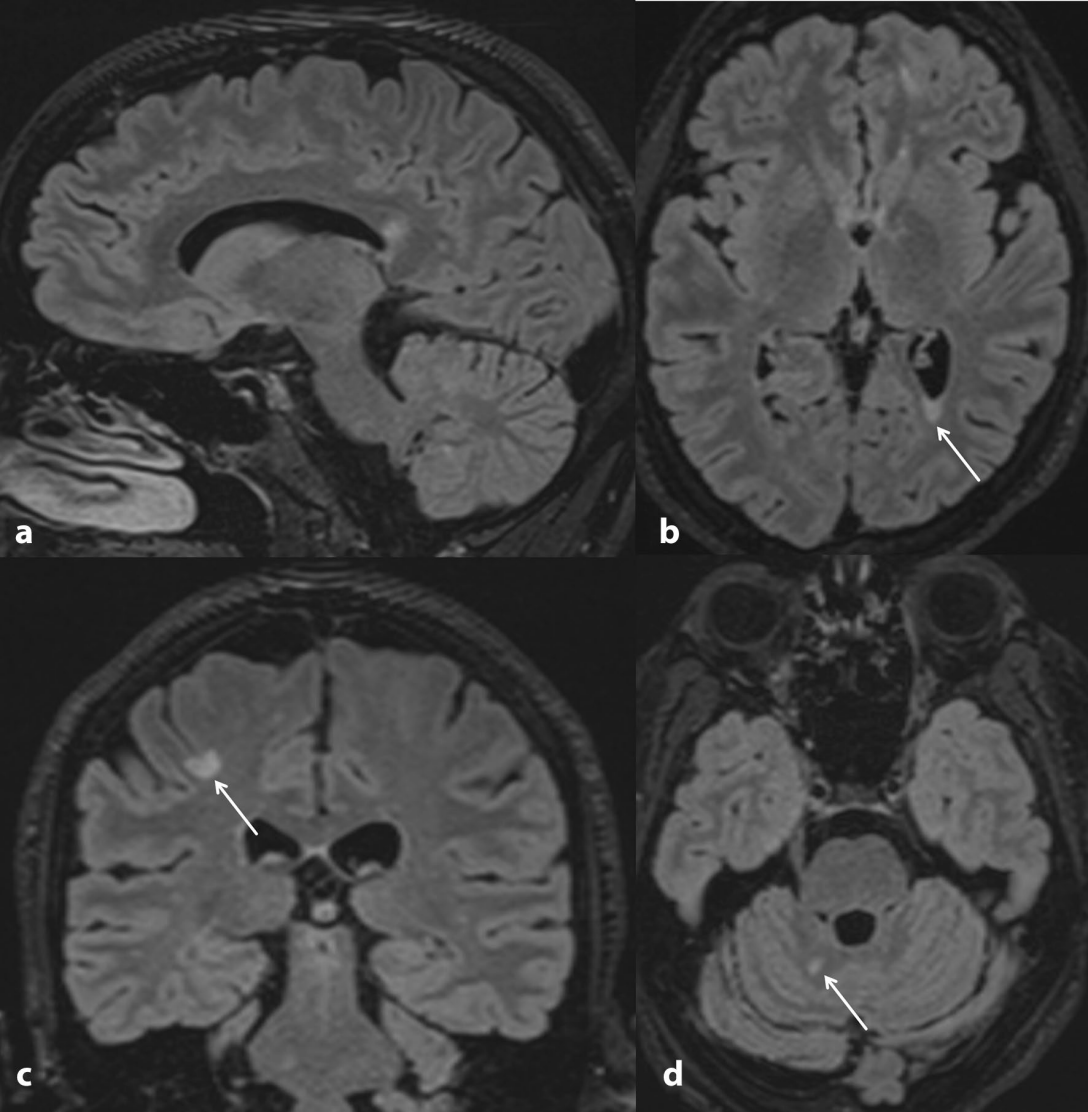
Cranial MRI performed one week after spinal MRI (Fig. 1). 3D FLAIR with 1 mm slice thickness and reconstruction in three planes. a The sagittal image shows a lesion in the splenium of the corpus callosum. b Axial image shows a periventricular lesion with triangular configuration. c Coronal image depicts a juxtacortical lesion involving the U-fibers. d Axial image shows involvement of the cerebellum. Overall, the MRI showed more than 20 specific lesions larger than 3 mm at periventricular, cortical/juxtacortical, or infratentorial locations without contrast enhancement
